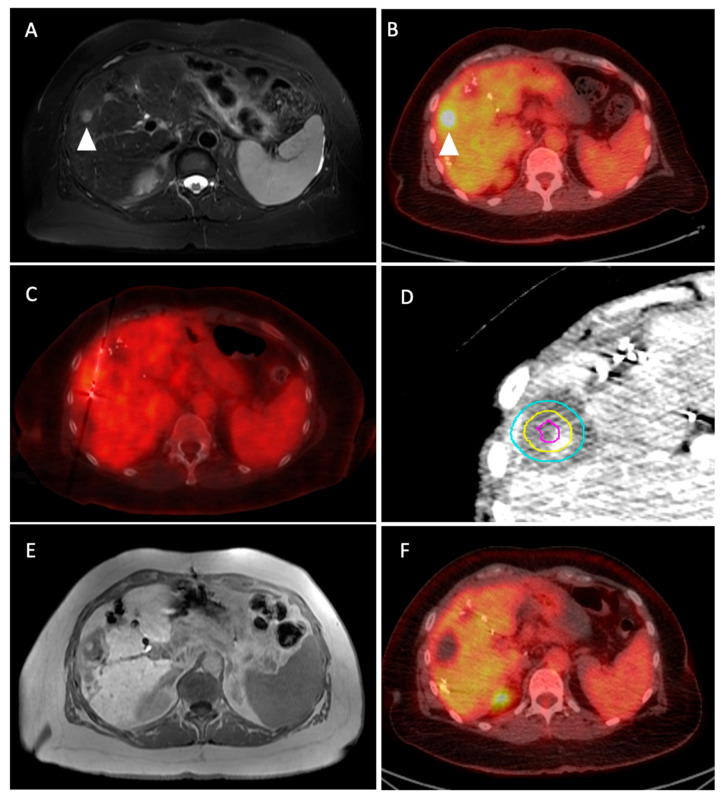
Sixty-one-year-old woman with metastatic colon cancer, status post chemotherapy, partial colectomy, hepatic arterial infusion pump chemotherapy and hepatic resection with intra-operative ablations, with new 1.2 cm segment 5 T2 hyperintense ((A): arrowhead)) FDG-avid (B) metastasis. This was treated with PET/CT-guided microwave ablation (C). The tumor was segmented on the immediate pre-ablation portal venous-phase CT, and subsequently registered to the post-ablation portal venous-phase CT ((D); purple border: segmented tumor, yellow border: 5 mm margin, light blue border: 10 mm margin), showing coverage of the tumor with adequate margins. Follow-up imaging after 6 weeks shows the segment 5 ablation zone without evidence of residual tumor ((E): hepatobiliary phase of MRI), with corresponding photopenic defect on concurrent PET/CT (F).
